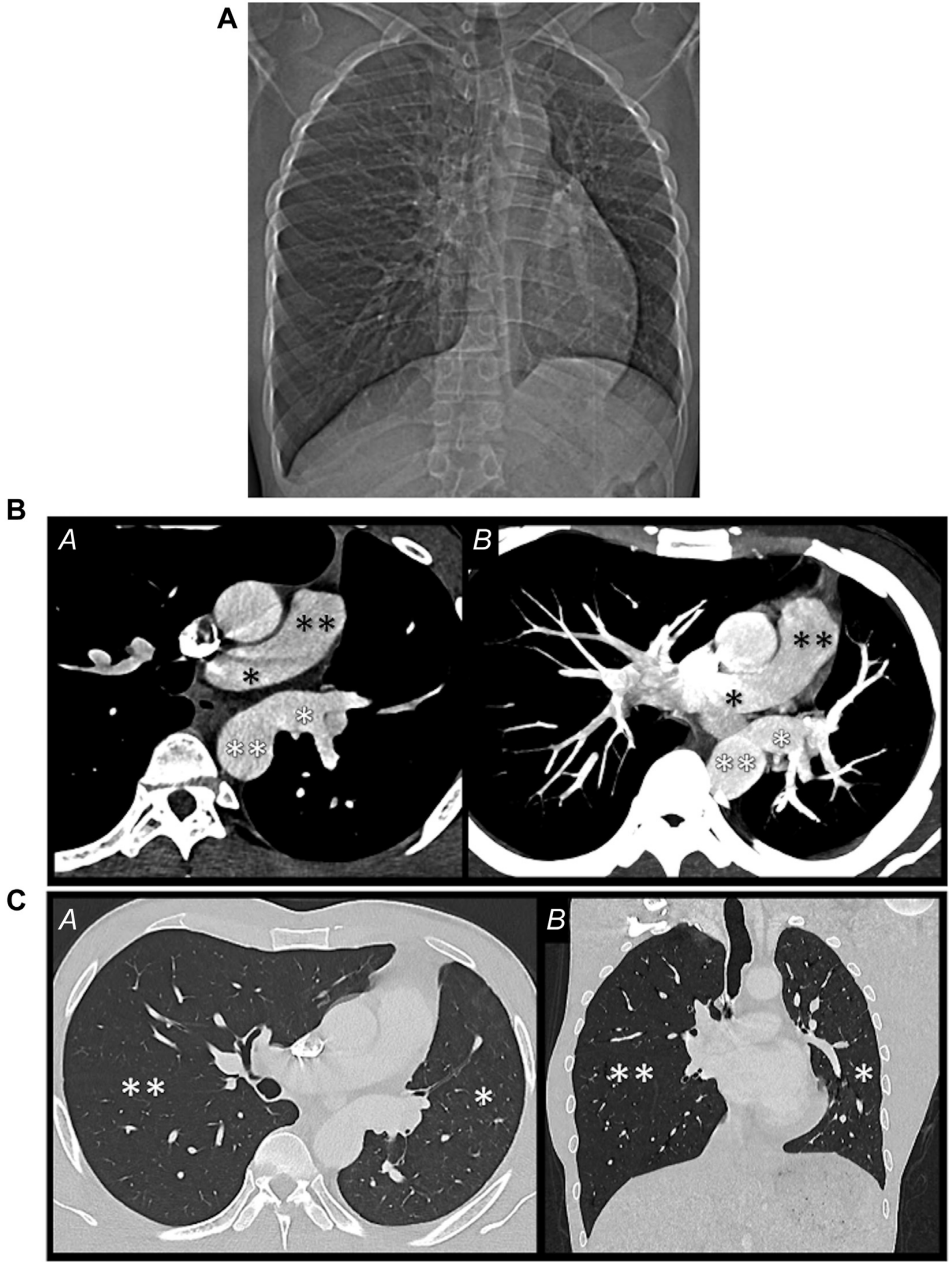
Computed Tomography Angiography (A) Scout view showing hypoplasia of the left lung and hyperinflation of the right lung. (B) Axial computed tomography angiography in the mediastinal window, from *(A)* top to *(B)* bottom, showing the right pulmonary artery (single black asterisk) and the pulmonary trunk (double black asterisk) and the left pulmonary artery (single white asterisk) from the descending thoracic aorta (double white asterisk). (C) *(A)* Axial and *(B)* coronal computed tomography angiography in the lung window showing hypoplasia of the left lung (single asterisk) and hyperinflation of the right lung (double asterisk).
